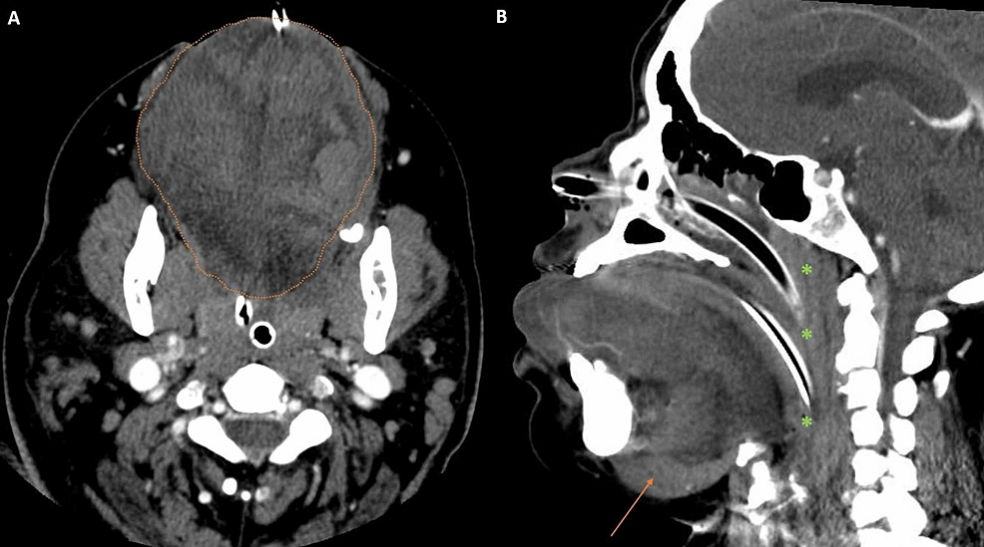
Axial and sagittal enhanced CT A: Axial enhanced CT demonstrating severe oral tongue swelling (orange outline). B: Sagittal enhanced CT showing oral tongue protruding through the oral cavity anteriorly and inferiorly displacing the mylohyoid (orange arrow). Edema of pharyngeal mucosa causing obstruction of naso- and oropharynx (green asterisks) is also seen CT: computed tomography
